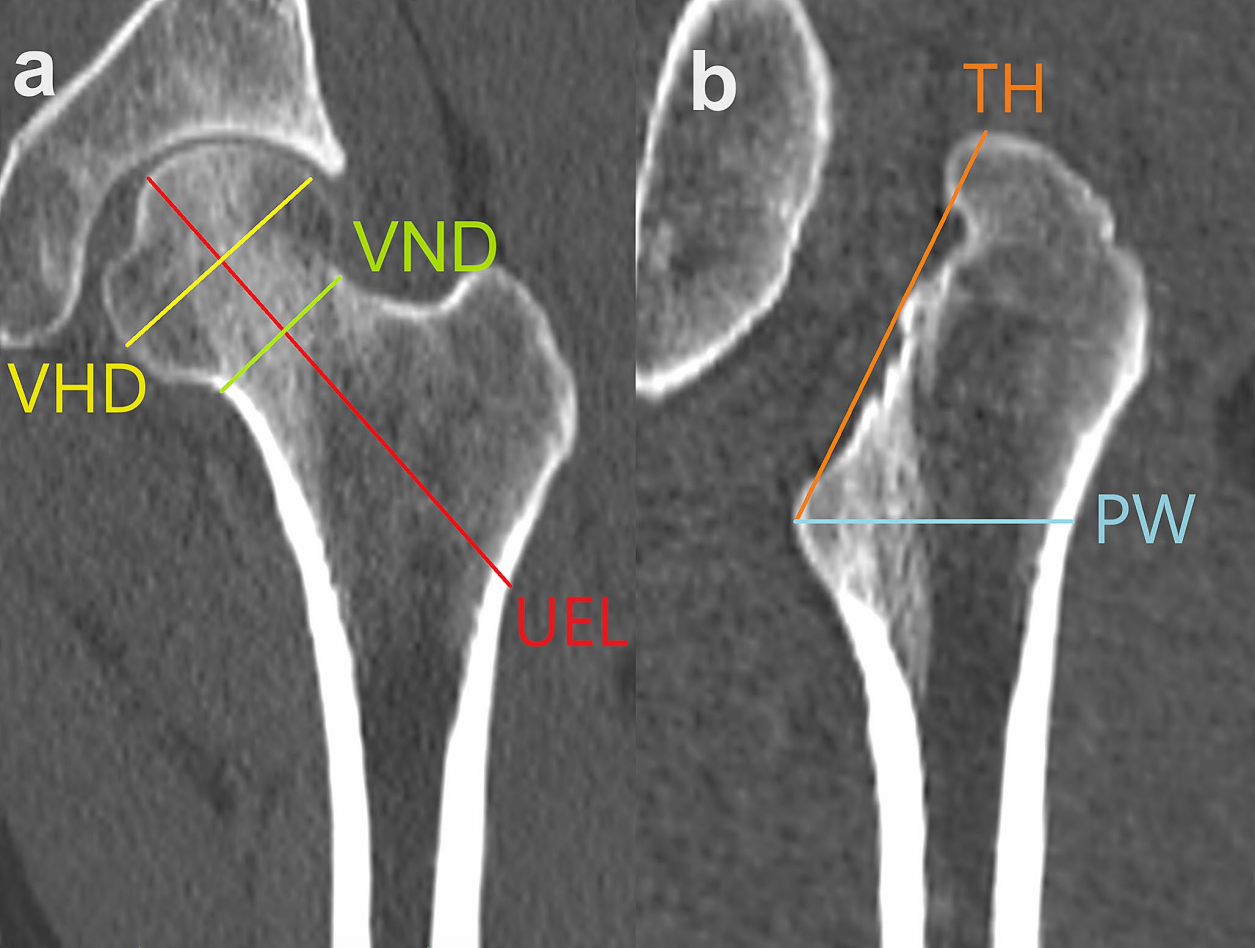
Two-dimensional computed tomography images showing five femoral measurements (see Table 1 for definition): (a) Upper epiphyseal length (UEL), vertical head diameter (VHD), and vertical neck diameter (VND) on a coronal oblique plane; (b) trochanter height (TH) and proximal width (PW) on a plane passing through PW and the superior point of the greater trochanter
